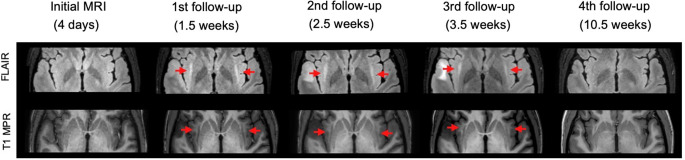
Evolution of the bilateral claustrum sign across serial MRI scans. The sign was absent on the initial MRI (4 days post-event), became apparent at the first follow-up MRI (1.5 weeks post-event), and persisted through the second and third follow-up MRI (2.5 and 3.5 weeks). It was no longer visible at the final follow-up MRI (10.5 weeks)
